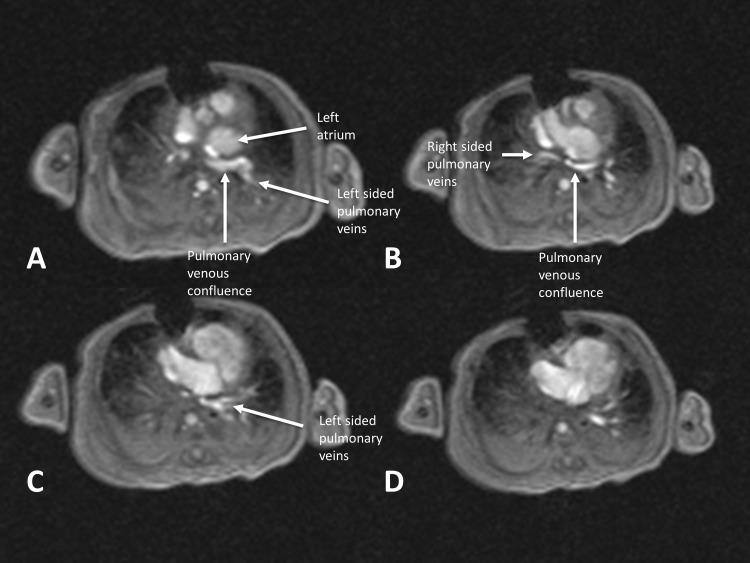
Anomalous pulmonary venous connection Magnetic resonance imaging from a single patient using a fast low angle shot (FLASH) imaging sequence in the superior to inferior direction that demonstrates totally anomalous pulmonary venous connections, with all four veins draining into a confluence posterior to the left-sided atrium.
